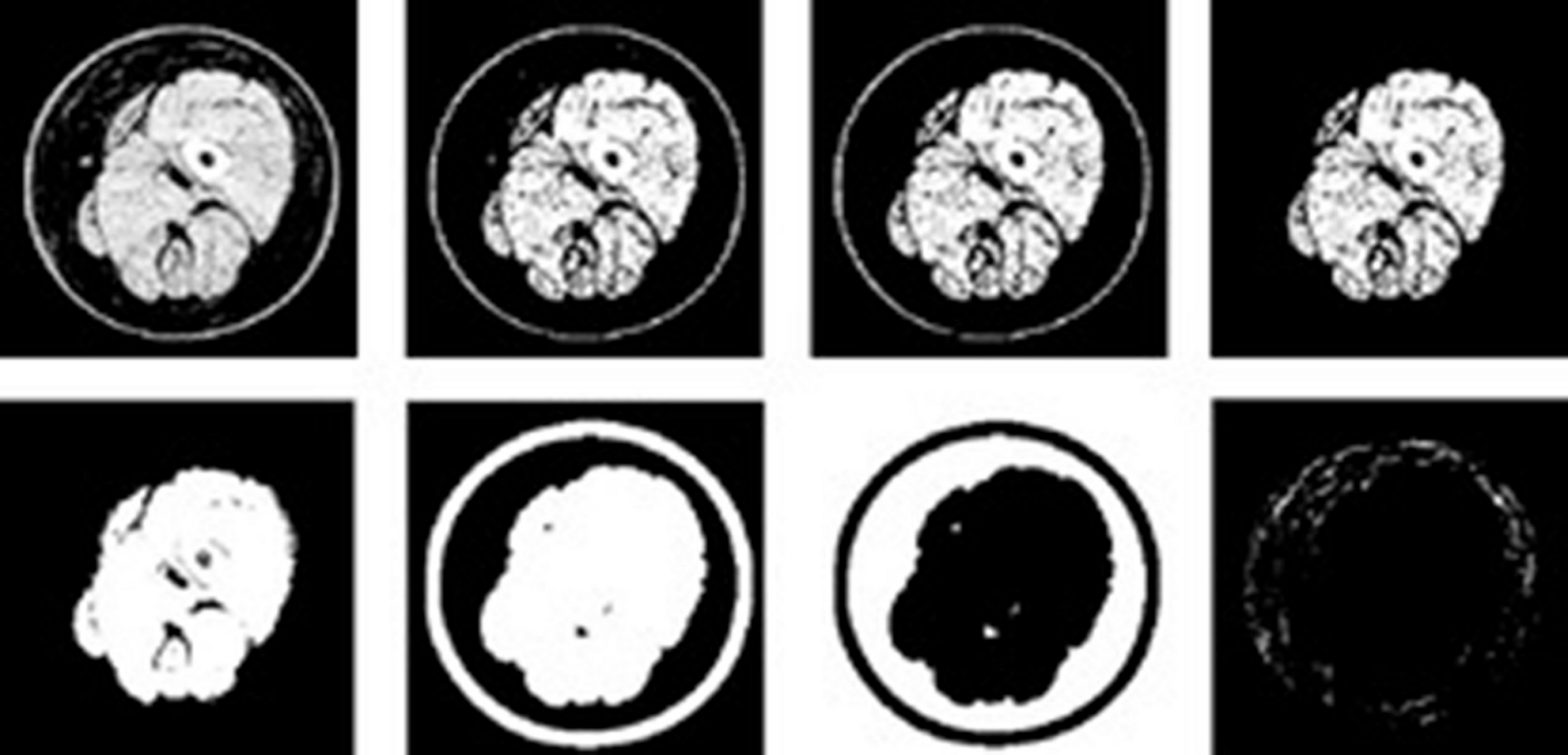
The images under the proposed image processing procedures. The original CT image of the affected limb (the first image; the farthest left one in the upper row), six intermediate images and the final image (farthest right in the lower row) are shown. The algorithm eliminated pixels from -50 Hounsfield unit (HU) to 26 HU (image 2), and removed any remained noise using a simple opening filter and manual reoperation (image 3). The outmost skin was removed based on its location (image 4). The remained pattern was processed as one connected region using the closing filter (image 5). Outside of this one connected region, the eliminated skin line was added as one outside thick line (image 6). This merged morphological pattern was converted by the black-white reversion operation. (image 7). The inversed pattern subtracted from the original CT image yielding a donut-shaped region between the skin and the muscle. (image 8). The nonblack pixels of the final image were regarded as epifascial lymph-proliferative fibrosis.
